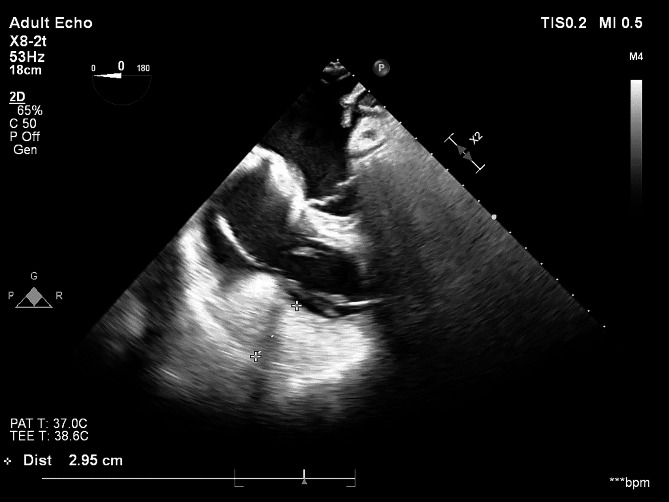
Transoesophageal echocardiography showing right ventricle myocardial thickening with free-wall of 30 mm
